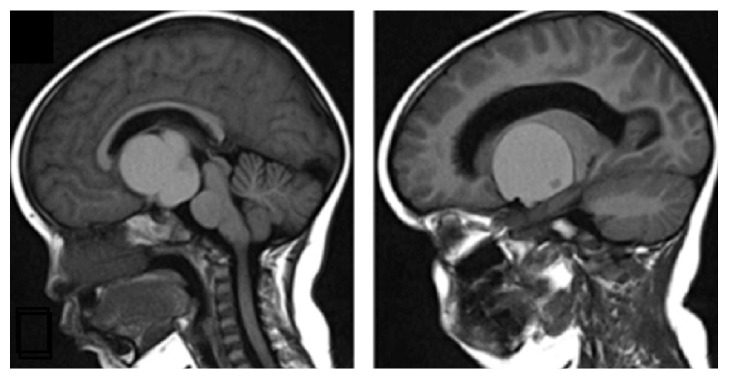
Pretreatment image. Initial Cerebral T1 weighted Magnetic Resonance Imaging showing large cystic suprasellar lesion.
